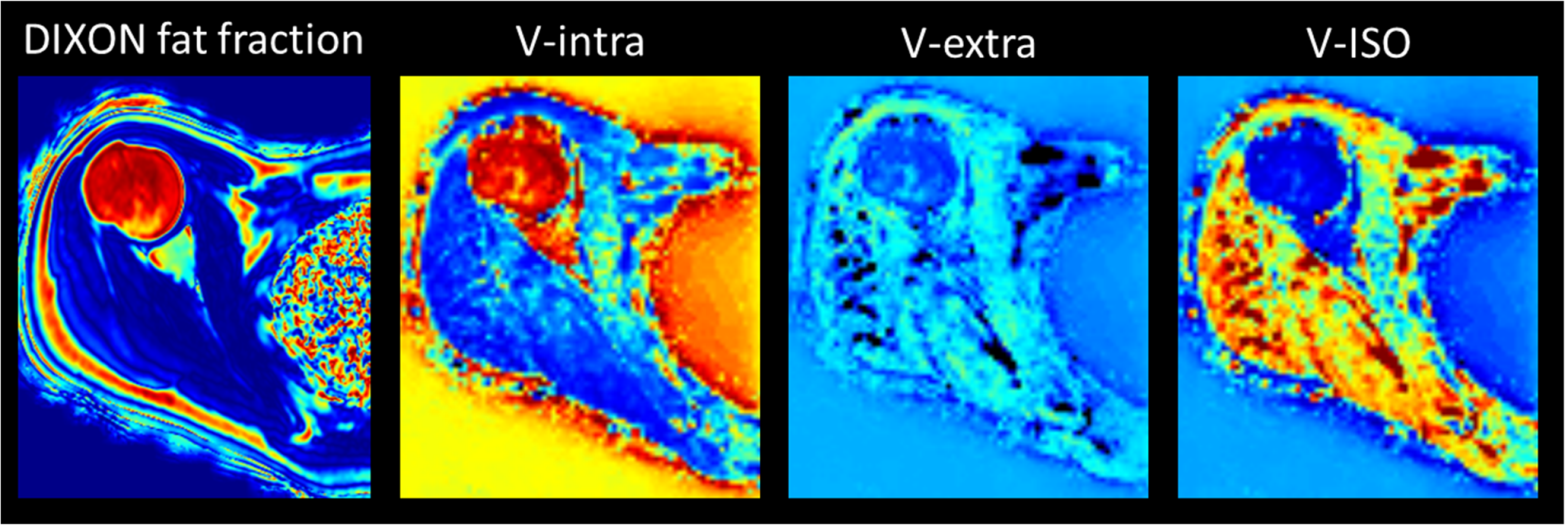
Exemplary representation of the acquired quantitative MRI sequences in a 25-year-old female
